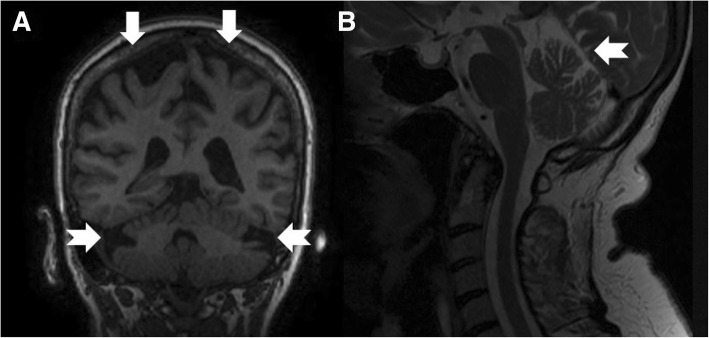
Coronal T1-weighted (a), mid-sagittal T2-weighted (b) of patient 5 at age 76. Note atrophy in the bi-parietal brain regions (thick arrows) as well as lateral hemispheres and superior vermis of the cerebellum (notched arrows)
